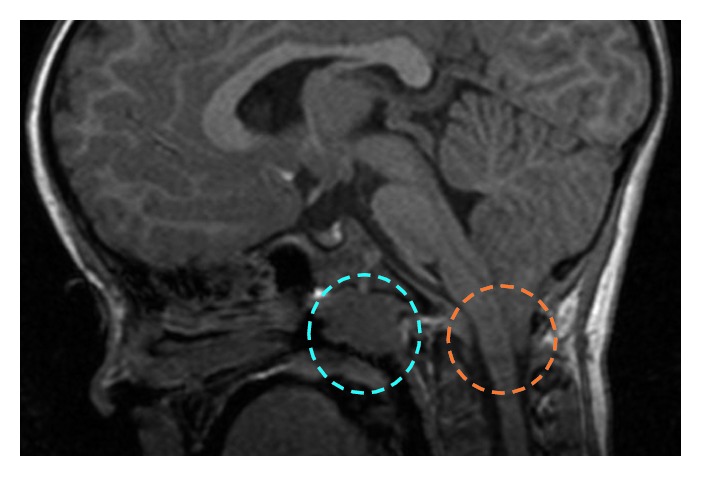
MRI of the brain shows Chiari malformation type 1 with herniation of the cerebellar tonsils beneath the foramen magnum of 17 mm and adenoid hypertrophy.
